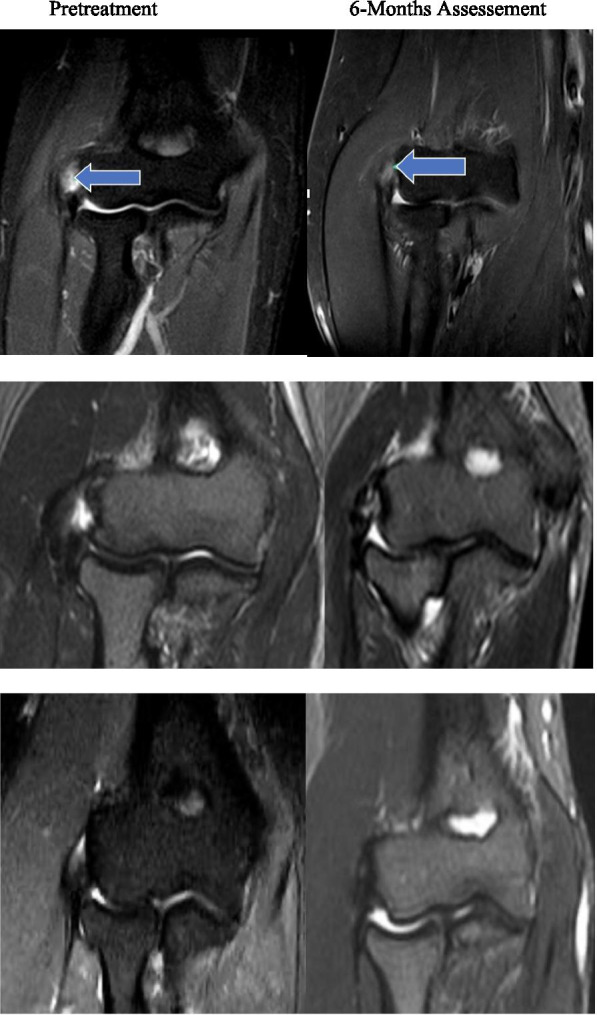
STIR Magnetic resonance imaging (MRI) results after autologous adipose derived stromal cells injection. Top row: patient 2´s score was 4 (tendinopathy [TD], 2; tear, 2) at pretreatment, and 3 (TD, 2; tear, 1) at 6 months assessement. Middle row: Patient 8´s score was 6 (TD, 3; tear, 3) at pretreatment, and 3 (TD, 2; tear, 1) at 6 months assessement. Botton row: Patient 17´s score was 3 (TD, 2; tear, 1) at pretreatment, and 2 (TD, 1; tear, 1) at 6 months assessement. Total MRI scores ranged from 2 to 6 (best to worst)
